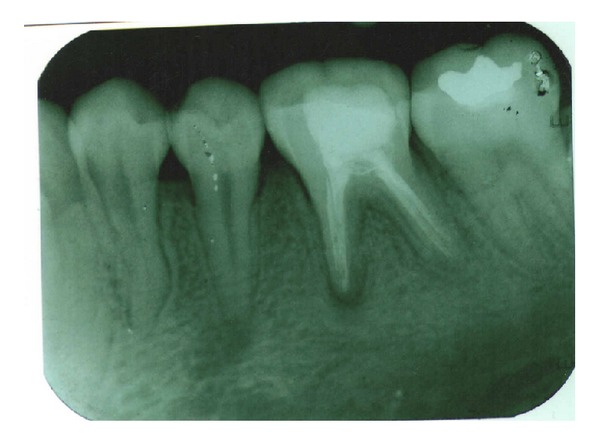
Radiograph showing healing after 9 months (46).
